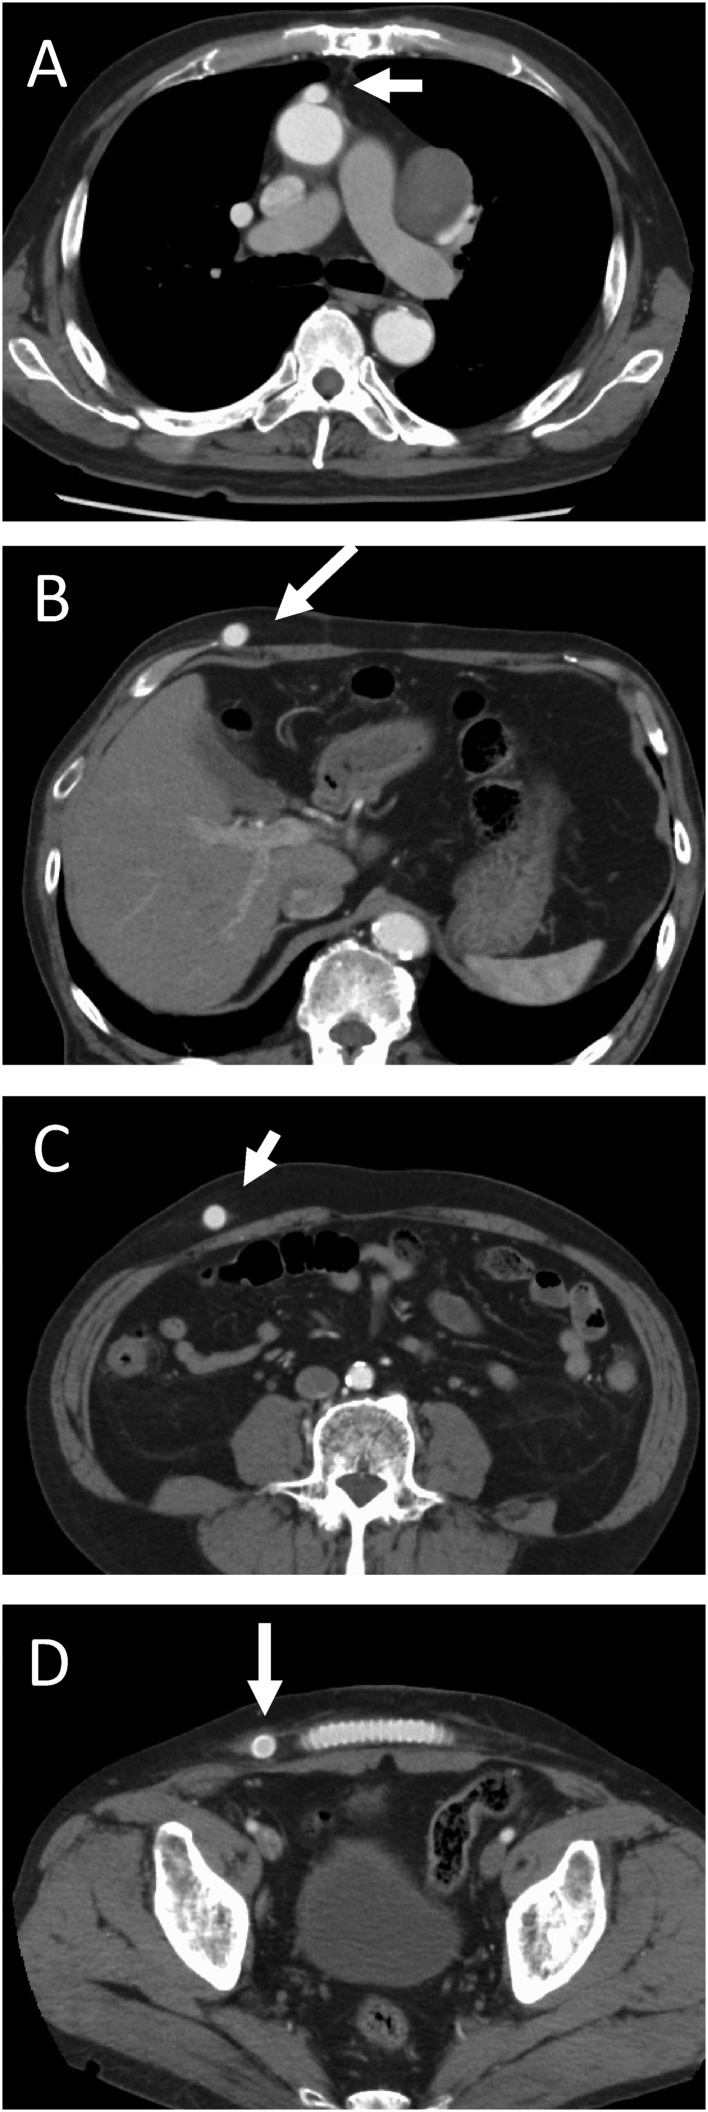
Computed tomography findings showed that graft was patency (white arrow).
